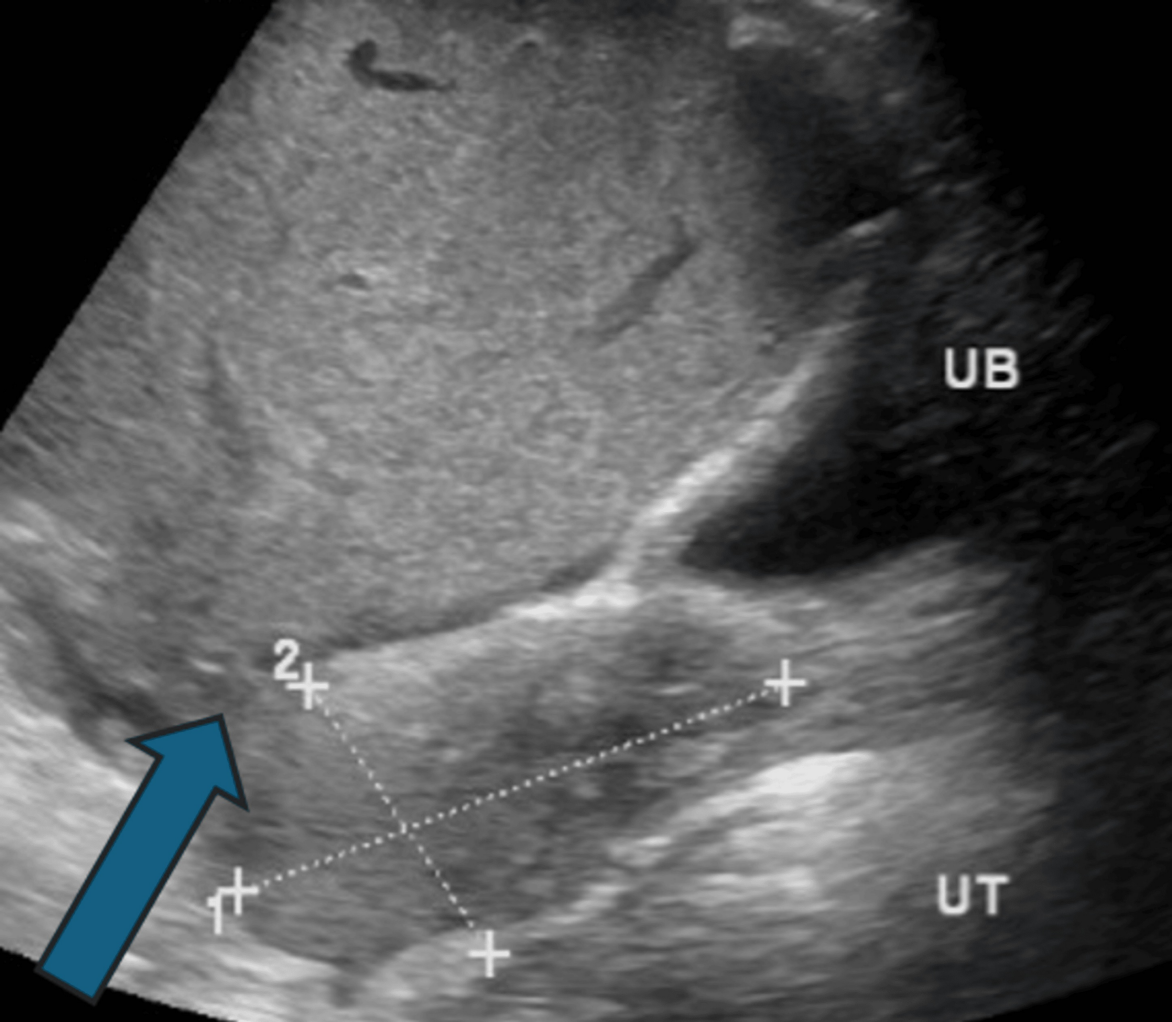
Ultrasound showing abnormal position of the spleen in the pelvis Blue arrow: spleen
